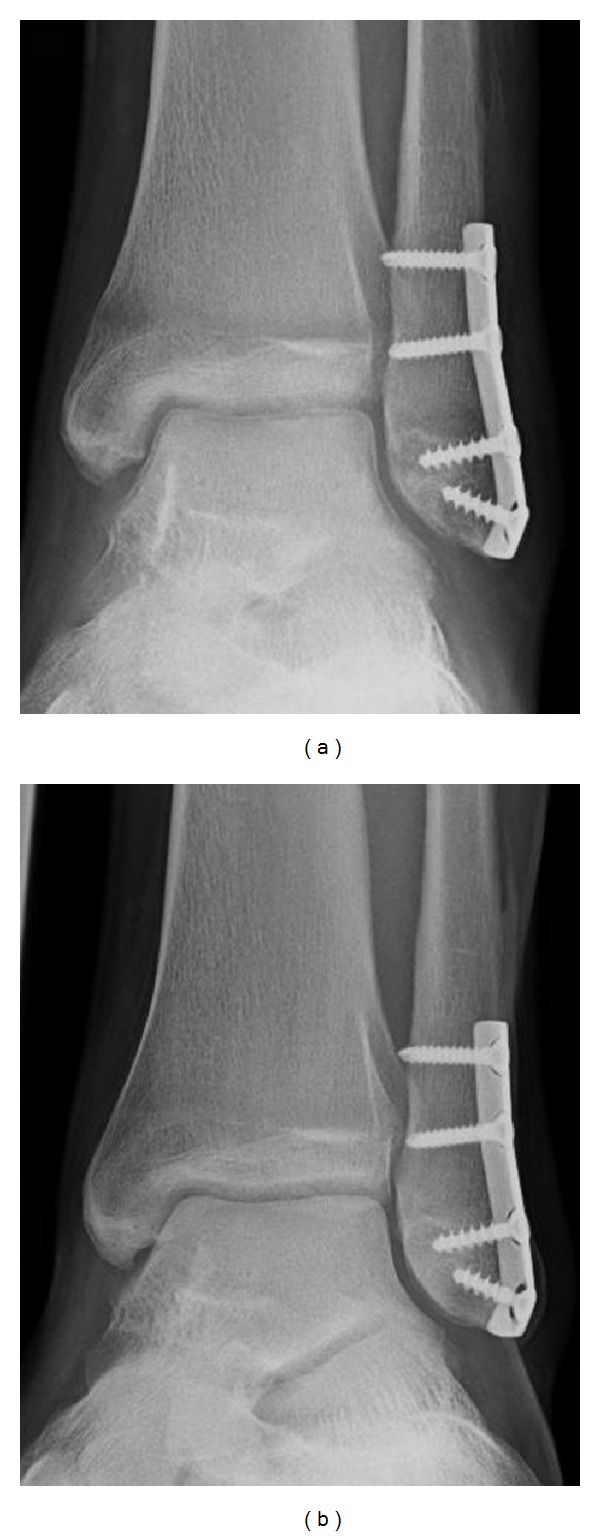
Serial radiographs of the left ankle after osteosynthesis of a distal fibula fracture. With immobility subchondral osteopenia of the talus as well as the distal tibial, epiphysis could be found (a) which disappeared after the resumption of normal activity (b).
